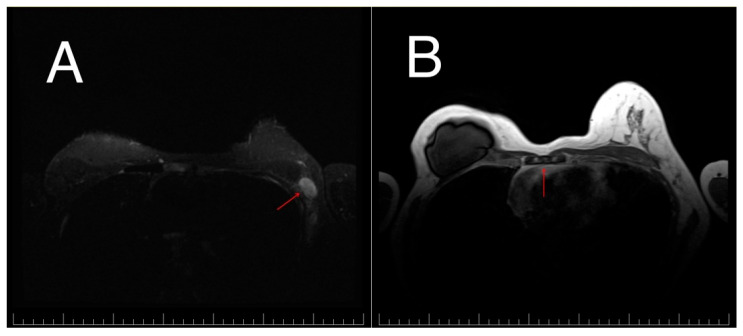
Axial contrast-enhanced breast MRI scan at the time of metastatic recurrence. (A) Left axillary lymphadenopathy. (B) Bone metastases involving the sternum.
